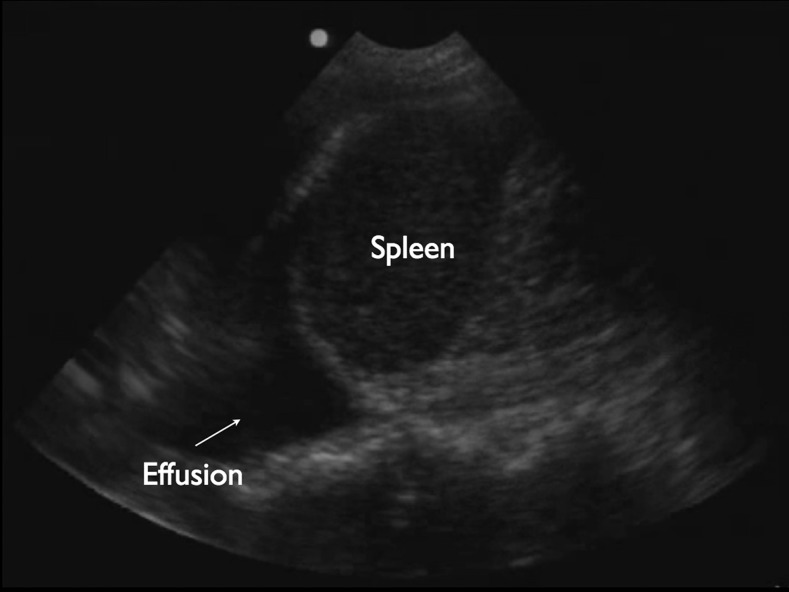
Ultrasound image of left pleural effusion. Simple pleural fluid appears black, or hypoechoic, on ultrasound in the costophrenic recess (arrow). Sonographic evidence of sub-clinical third-spacing of fluid in Dengue fever can be seen even without physical exam findings consistent with pleural fluid.
